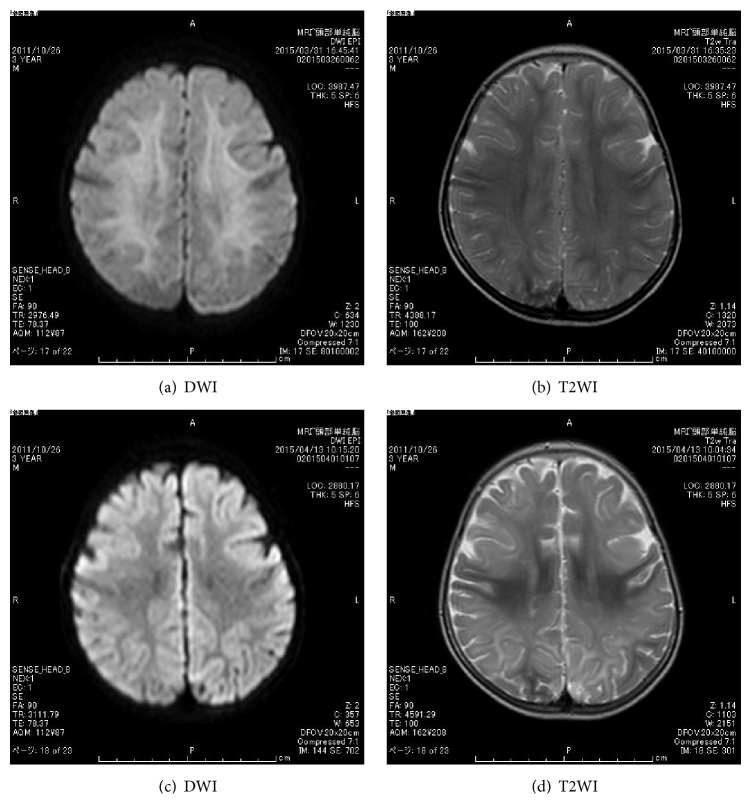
Magnetic resonance imaging (MRI) findings. Both diffusion-weighted imaging (DWI) ((a) and (c)) and T2-weighted imaging (T2WI) ((b) and (d)) were performed. MRI performed on day 8 showed hyperintensity in the deep subcortical white matter ((a) and (b)). The hyperintensity on DWI resolved (c), but diffuse atrophy was noted.
